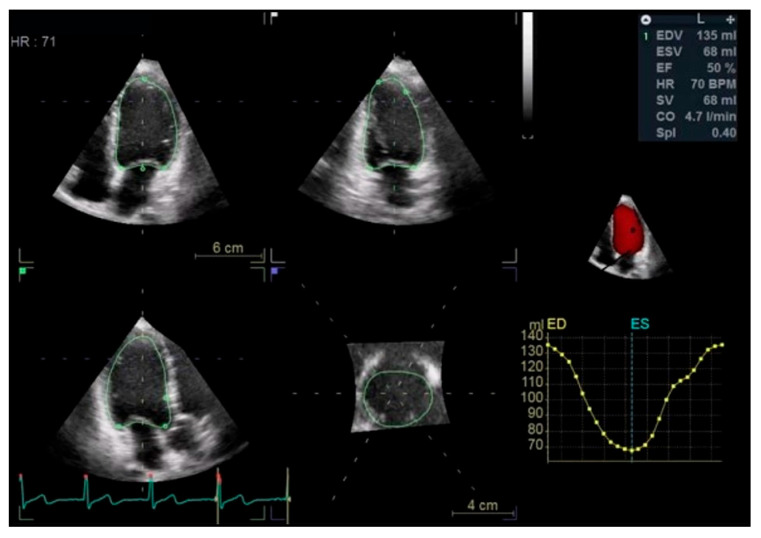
The assessment of left ventricular ejection fraction by 3D transthoracic echocardiography.
